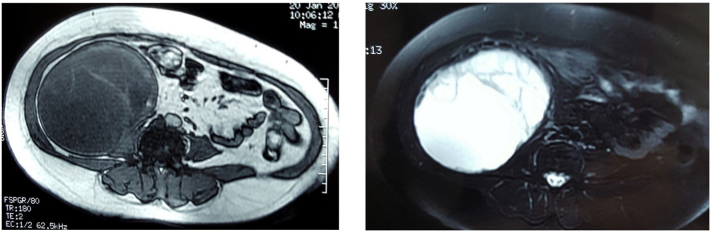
MRI confirming the cystic nature of the mass.
